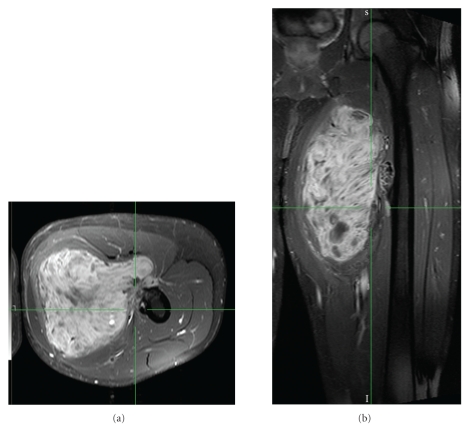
Axial (a) and coronal (b) magnetic resonance imaging scans of soft tissue sarcoma arising from the adductor muscle and invading the periosteum of the femur.
